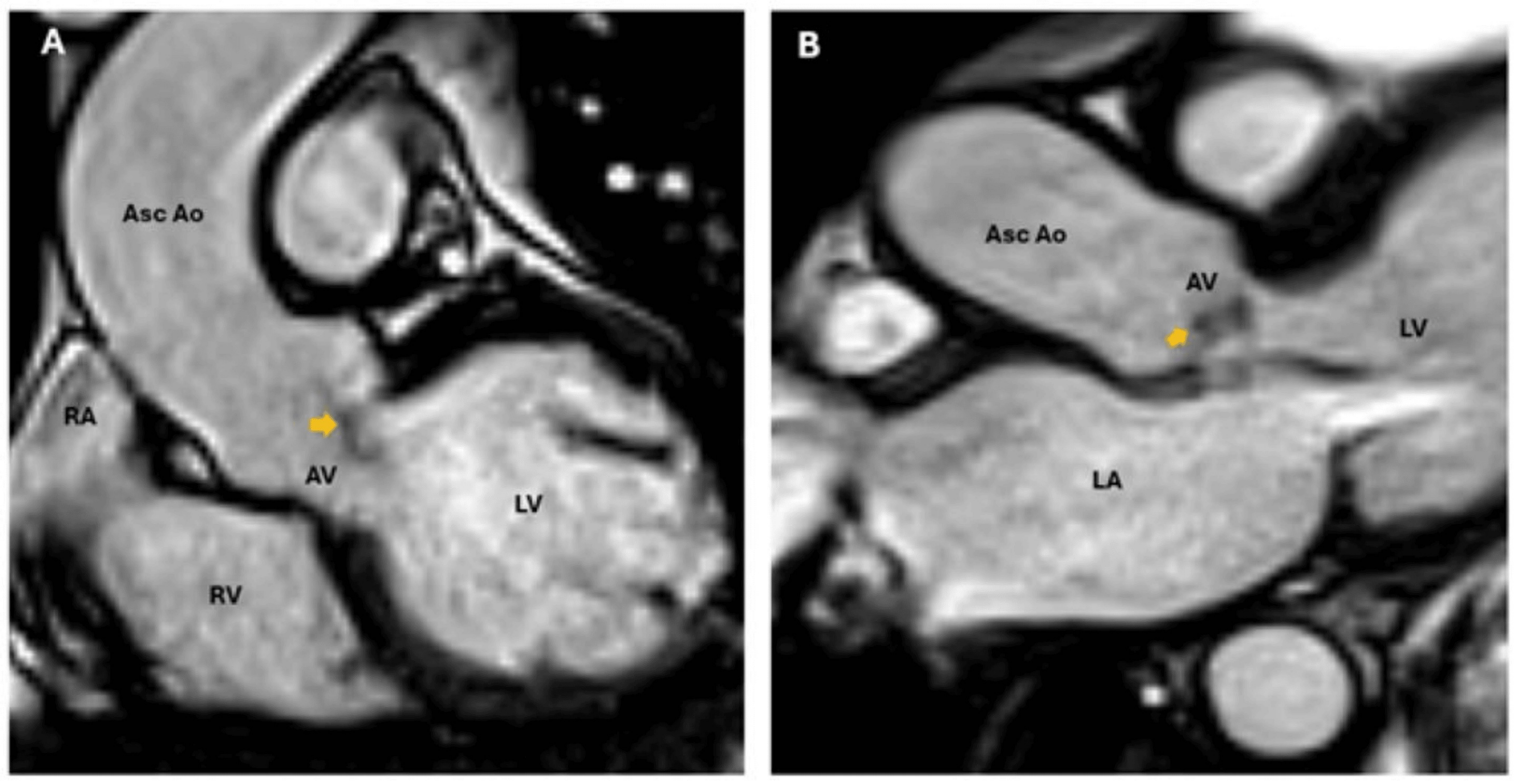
Cardiac MRI three-chamber views (Panels A and B) demonstrate an aortic valve with leaflet thickening and a vegetation adherent to one of the aortic cusps (yellow arrow). LA: left atrium RA: right atrium LV: left ventricle RV: right ventricle AV: aortic valve Asc Ao: ascending aorta
